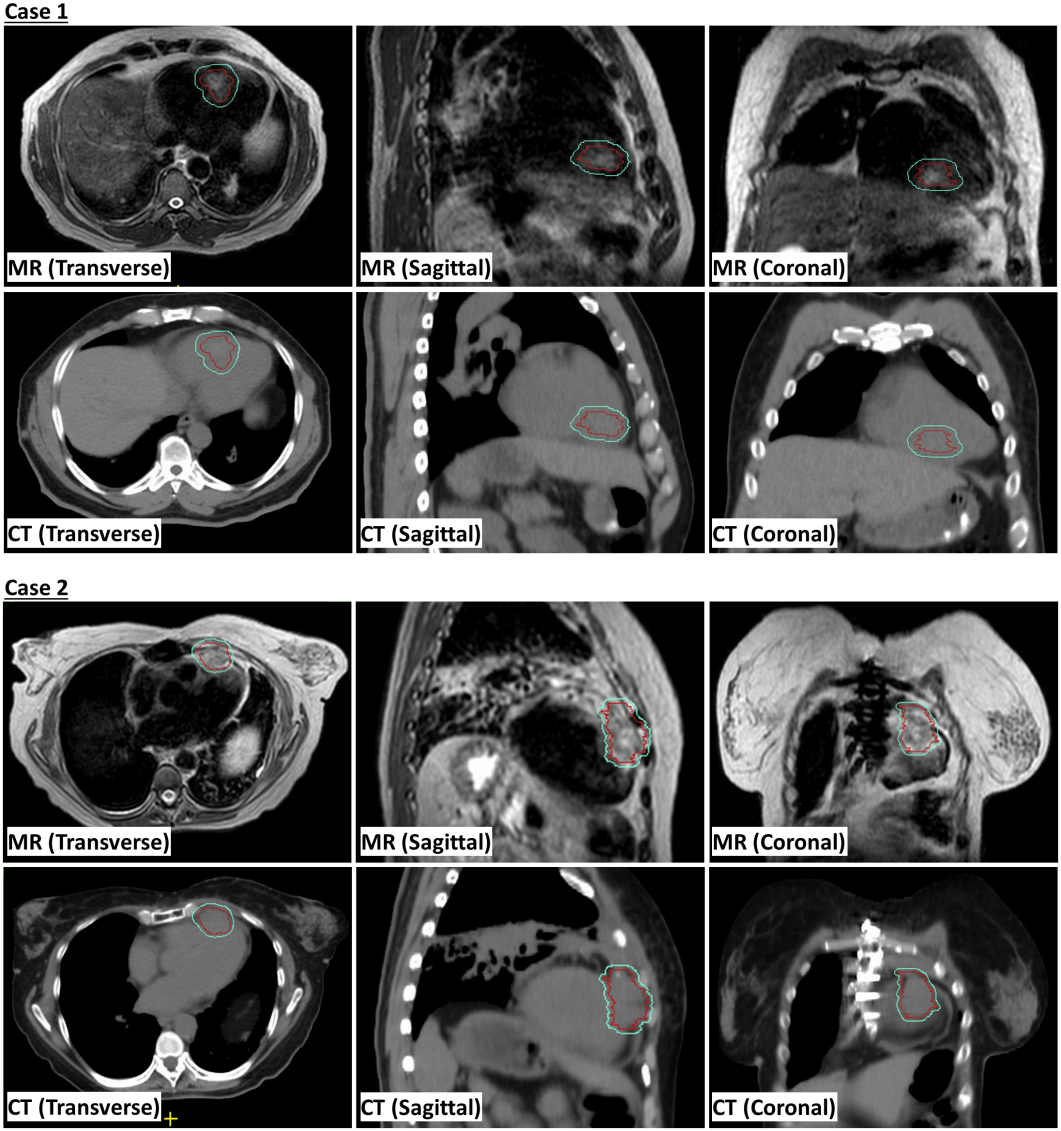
Magnetic resonance (MR) and computed tomography (CT) scans showing internal gross tumor volume (iGTV) in red and planning risk volume (PTV) in cyan.
